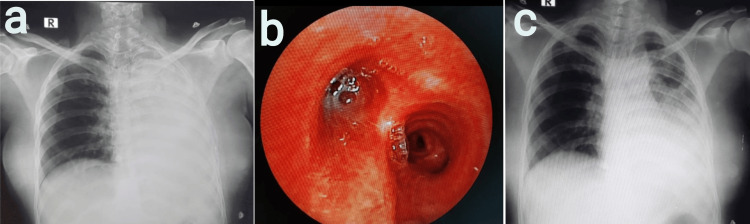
a) Chest radiograph revealed homogeneous opacities on the left side with a mediastinal shift to the left, b) bronchoscopy revealed left congested mucosa with large mucus plugs obscuring the left main bronchus, and c) chest radiograph revealed the expansion of the collapsed lung post bronchoscopy
